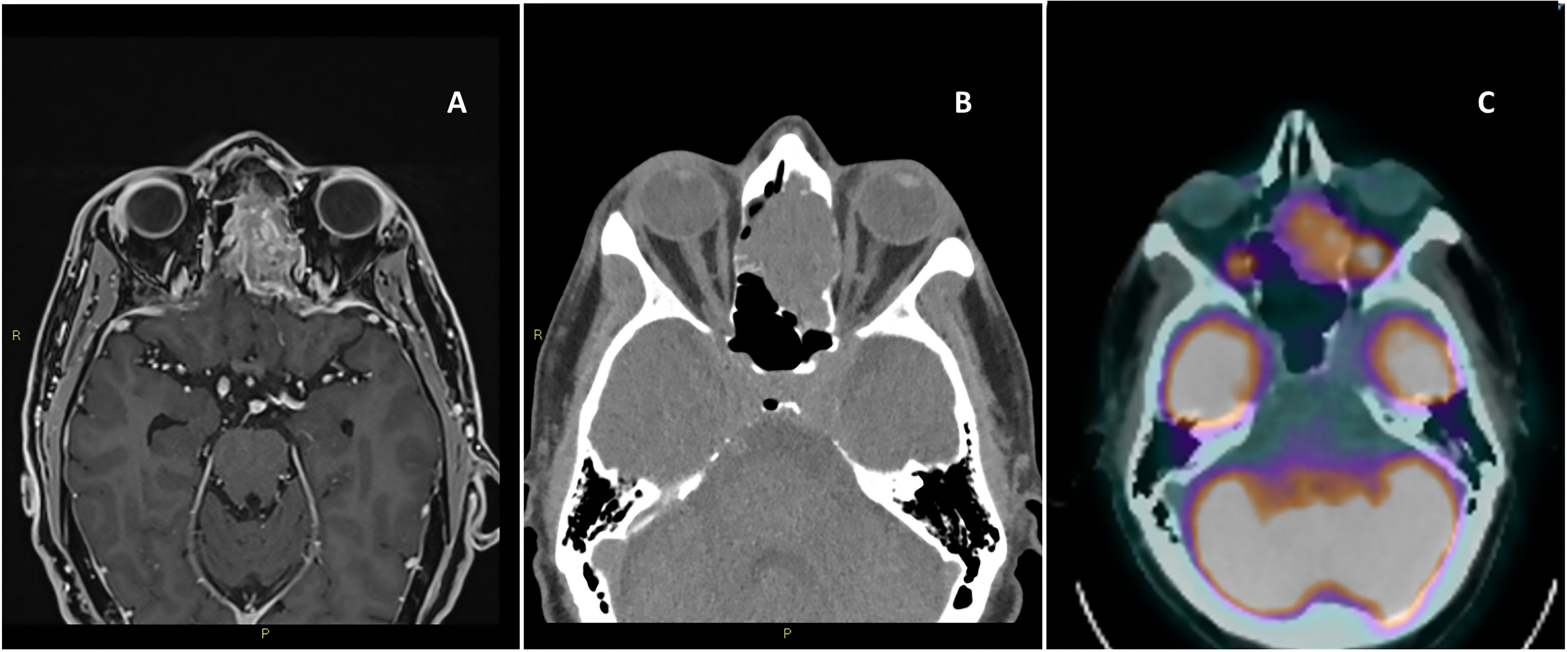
Magnetic resonance imaging of the brain T1 with contrast, axial view (A), computer tomography of the brain axial view without contrast (B), positron emission tomography axial view (C).
